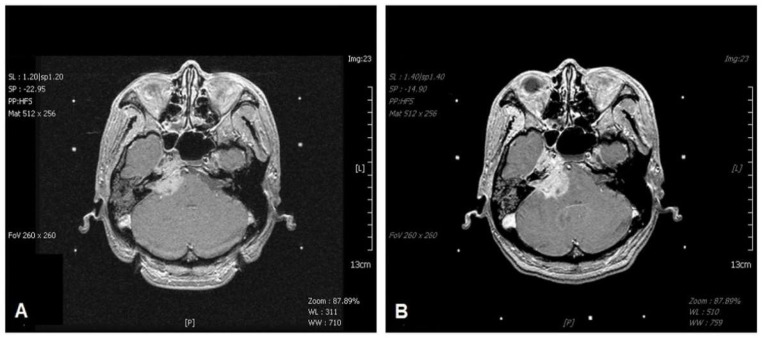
T1-weighted magnetic resonance imaging (MRI) of the brain revealing a well-enhanced tumor with extension to the right cavernous sinus and intradural invasion (T4b2) after gadolinium injection (A). The tumor was treated with GKS using a margin dose of 12 Gy. Six months later, the follow-up brain MRI reveals tumor progression (B).
